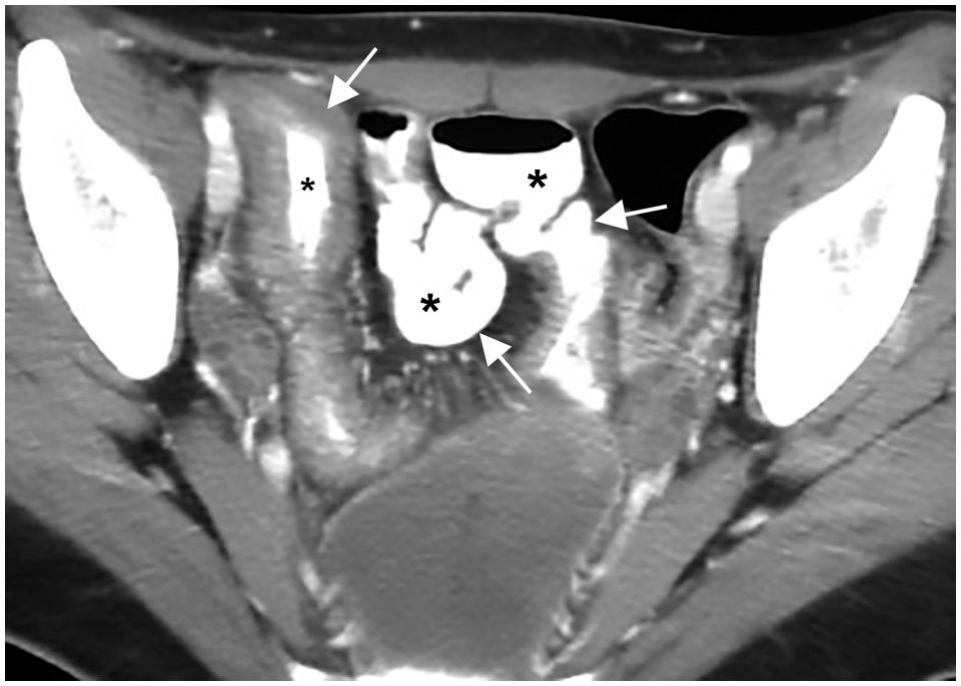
Abdomen CT in a patient with known Crohn’s disease. The examination, performed after iodinated contrast medium injection and orally administration of positive contrast medium (3% water-soluble iodinated solution), shows some pelvic ileal loops, which the lumen is enhanced by positive contrast agent (black asterisks). Hyperdensity of the bowel lumen hides the normal and pathological intestinal walls and the characteristics of their enhancement (white arrows).
